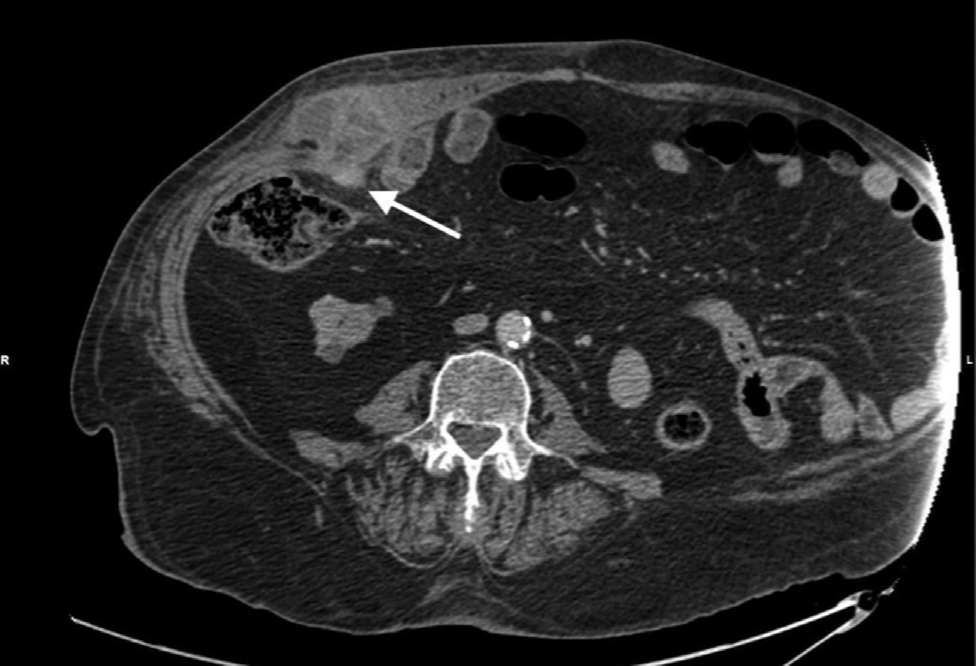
Computed tomography scan showing the tip of the appendix (arrow) protruding through the Spigelian hernia.
